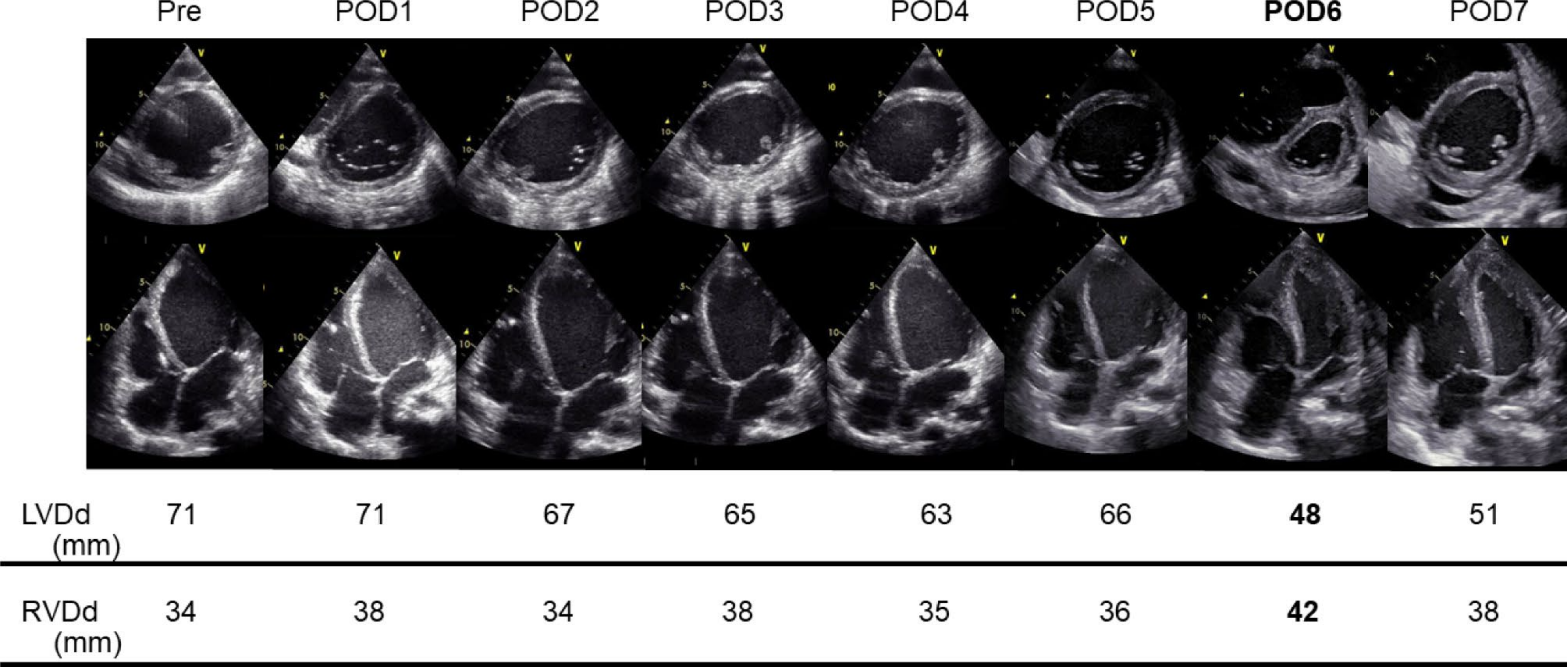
Daily echocardiographic findings during left ventricular assist device pump flow adjustment. These echocardiographic observations showed hemodynamic changes occurring at POD6, which revealed significant IVS shifting to the left ventricle. LVDd: left ventricular end-diastolic dimension, LVD: left ventricular diameter at end-systole, Pre: preoperative, POD: postoperative day
